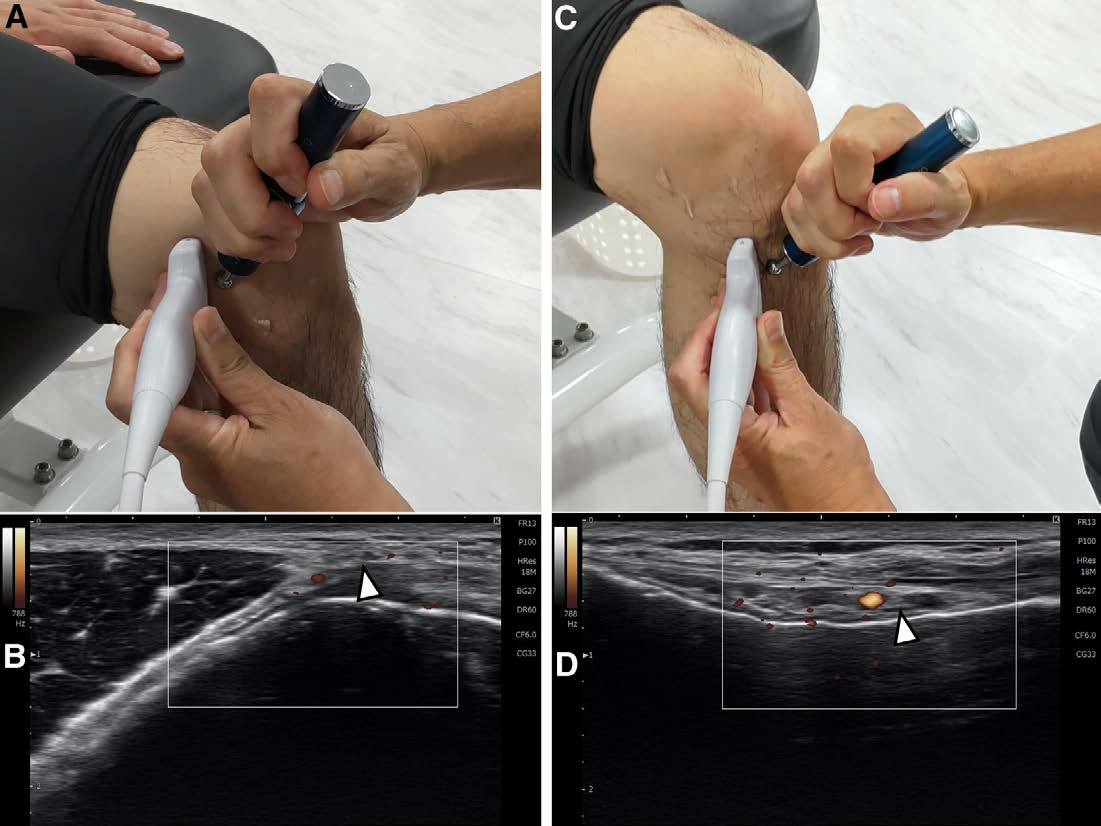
Genicular nerves are difficult to detect on ultrasound images, so arteries are used as an indicator. (A, B) The transducer placement is indicated for the cross-sectional view of the left superior medial genicular artery with a power Doppler image (white arrow) and (C, D) the left inferior medial genicular artery with a power Doppler image (white arrow).
